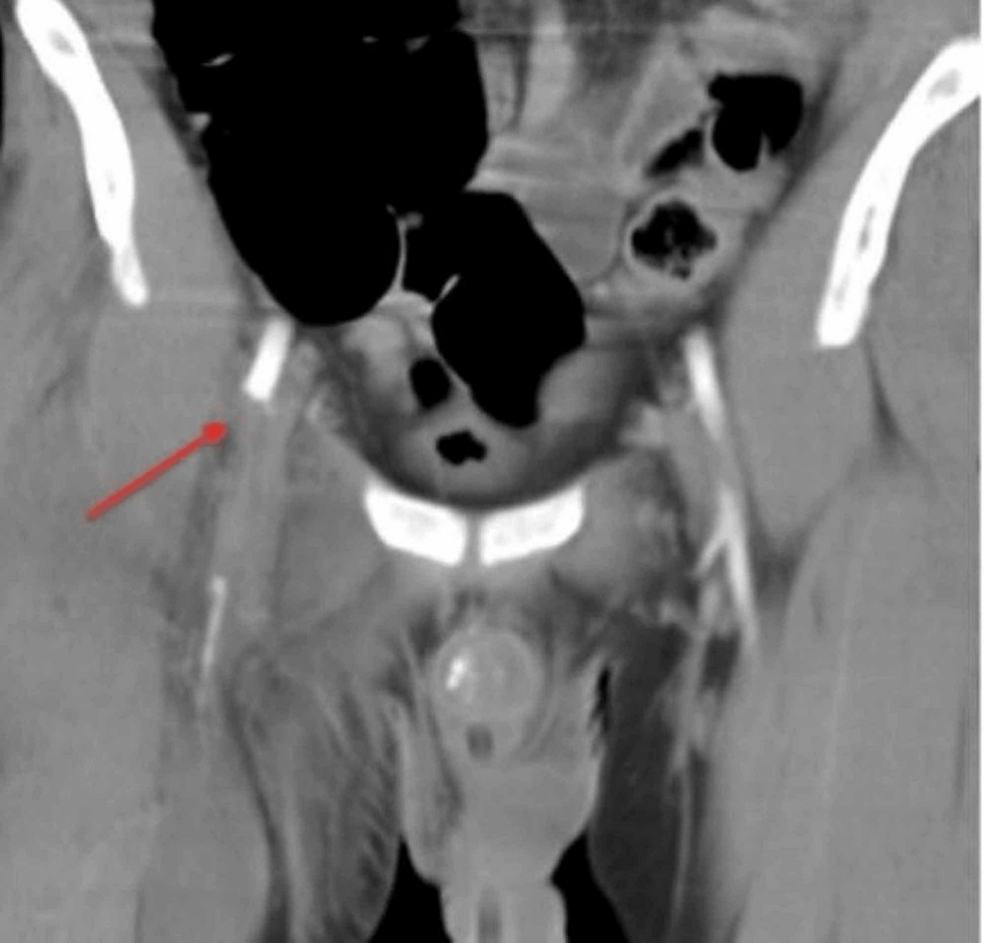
Computed Tomography Angiogram (CTA) showing 5 cm occlusion of the right common femoral artery (CFA) (red arrow).
